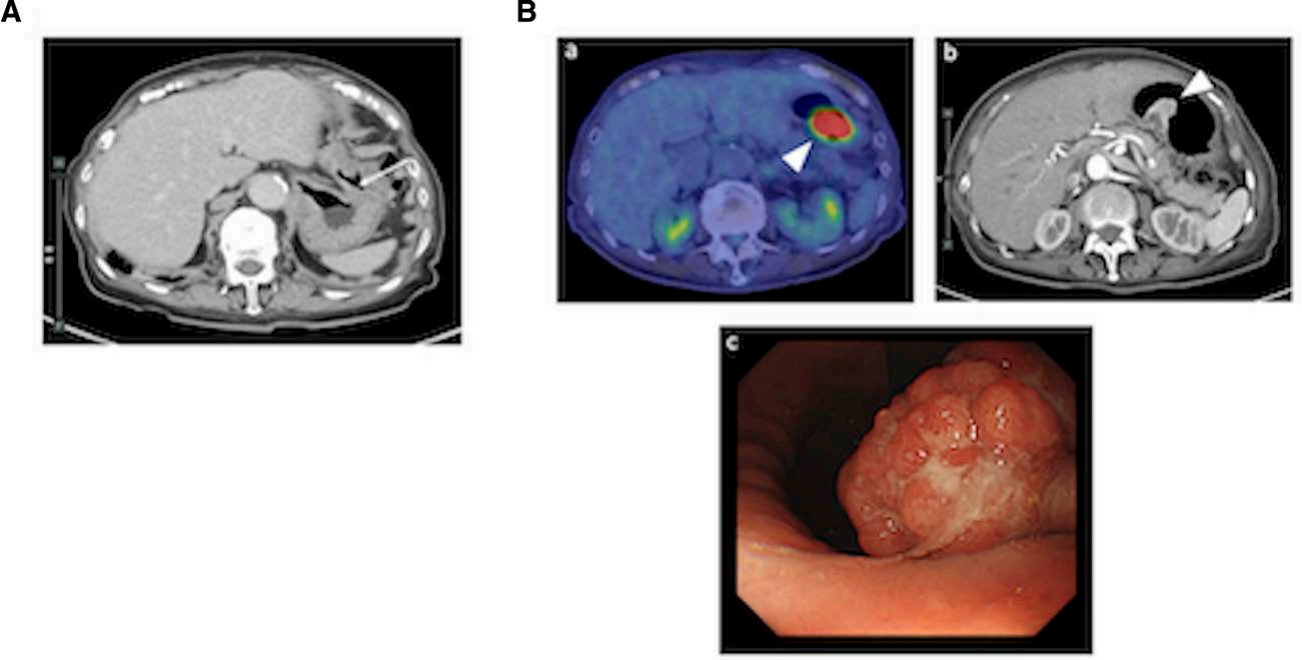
Imaging findings in gastric cancer during the clinical course. (A) Tumor in the angular incisure (arrow) is not evident on plain CT at the initial examination. (B) a. FDG-PET/CT performed 6 months later shows strong accumulation in the stomach. b. Contrast-enhanced CT reveals an elevated lesion consistent with the accumulation. c. Upper endoscopy shows advanced gastric cancer in the angular incisure, and biopsy confirms a diagnosis of adenocarcinoma.
